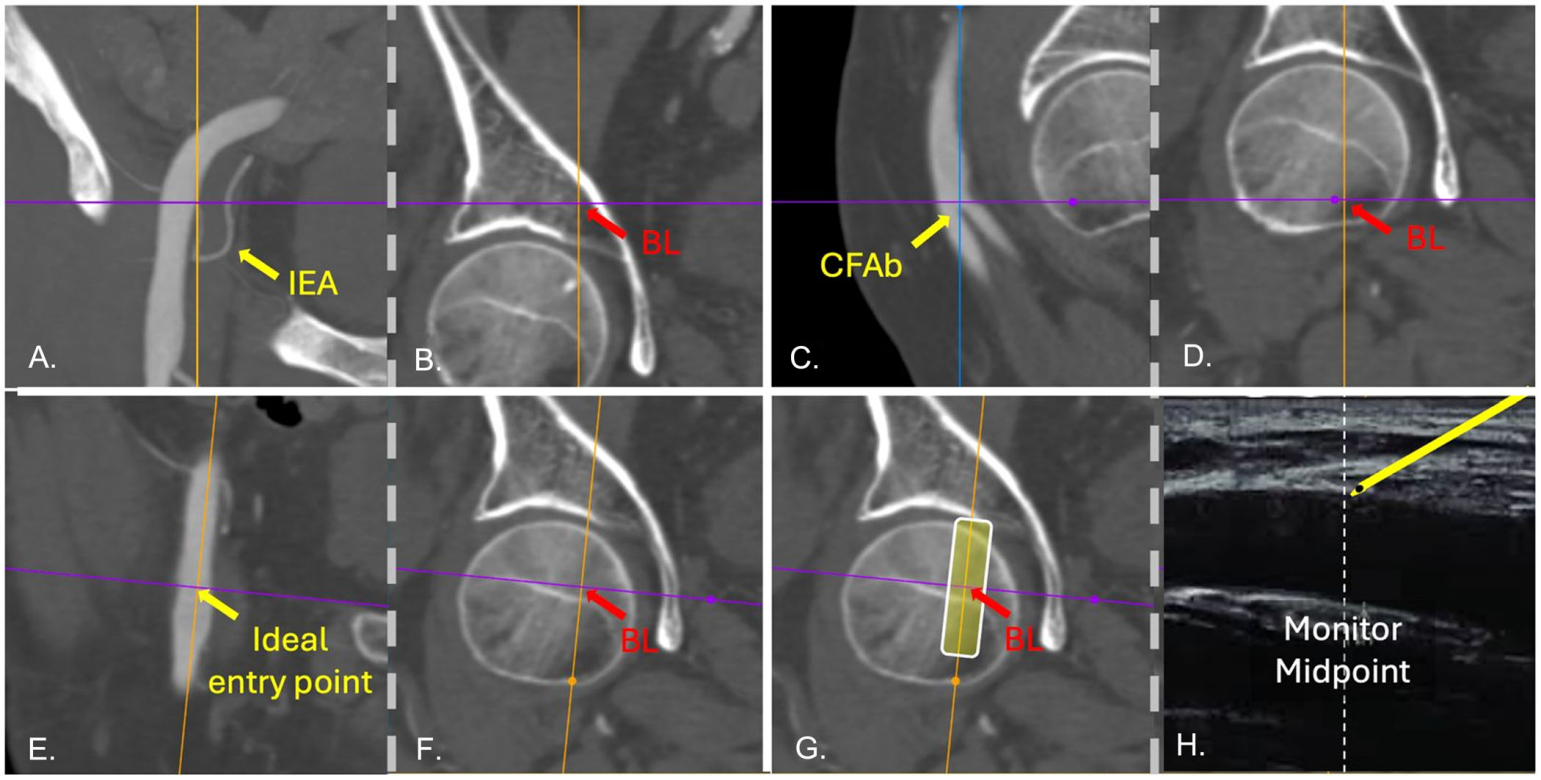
Multimodality imaging to aid ultrasound‐guided CFA puncture. (A) Positioning the cursor at the inferior epigastric artery (IEA) origin. (B) After scrolling the cursor at the coronal plane, the level of the IEA is marked in the femoral bone/acetabulum. (C) Positioning the cursor at the level of the common femoral artery bifurcation (CFAb) in the sagittal plane. (D) After scrolling the cursor at the coronal plane, the level of the CFAb is marked in the femoral bone. (E) The cursor is positioned at the level of the ideal entry point in the CFA. (F) After scrolling the cursor at the coronal plane. [Color figure can be viewed at wileyonlinelibrary.com]
